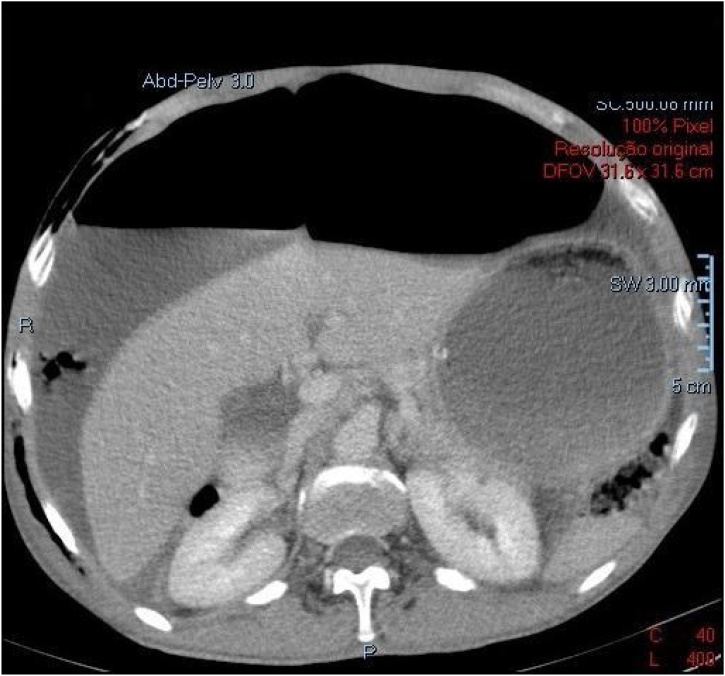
CT of Pneumoperitoneum after perforation (2018).
